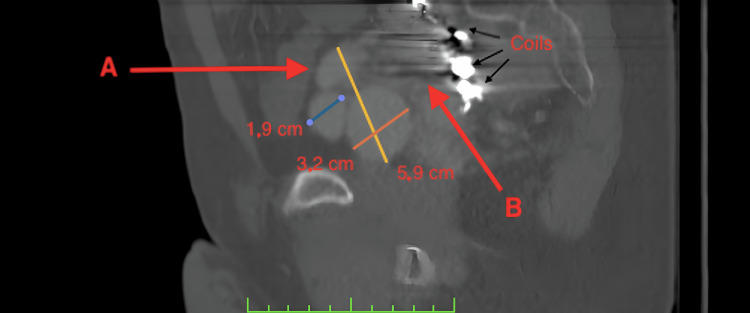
CT angiography. A sagital section of the pelvis. Note the endovascularly placed coils and the enormous right internal iliac vein (B), as well as the aneurysmatic venous confluent branch (A) two years after endovascular therapy.
